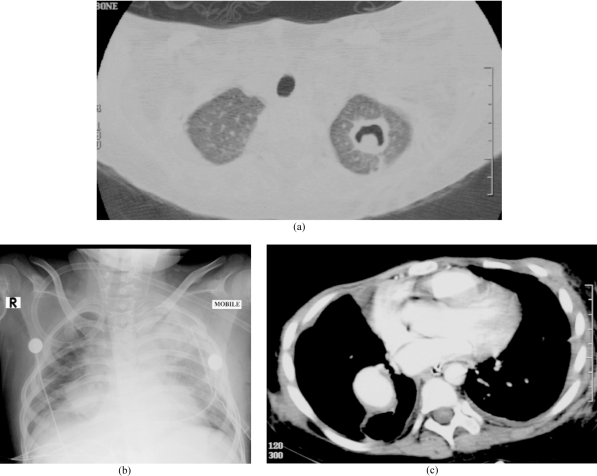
A 10-year-old girl with leukemia and biopsy proven Aspergillus infection of the lung. She later developed a mycotic aneurysm in the chest. (a) CT showed a cavity with a “crescent sign” in keeping with an Aspergilloma. The lesion was excised. (b) Chest radiograph two months later showed a round opacity in the right lung. (c) CT revealed an aneurysm of the lower lobe branch of the right pulmonary artery.
